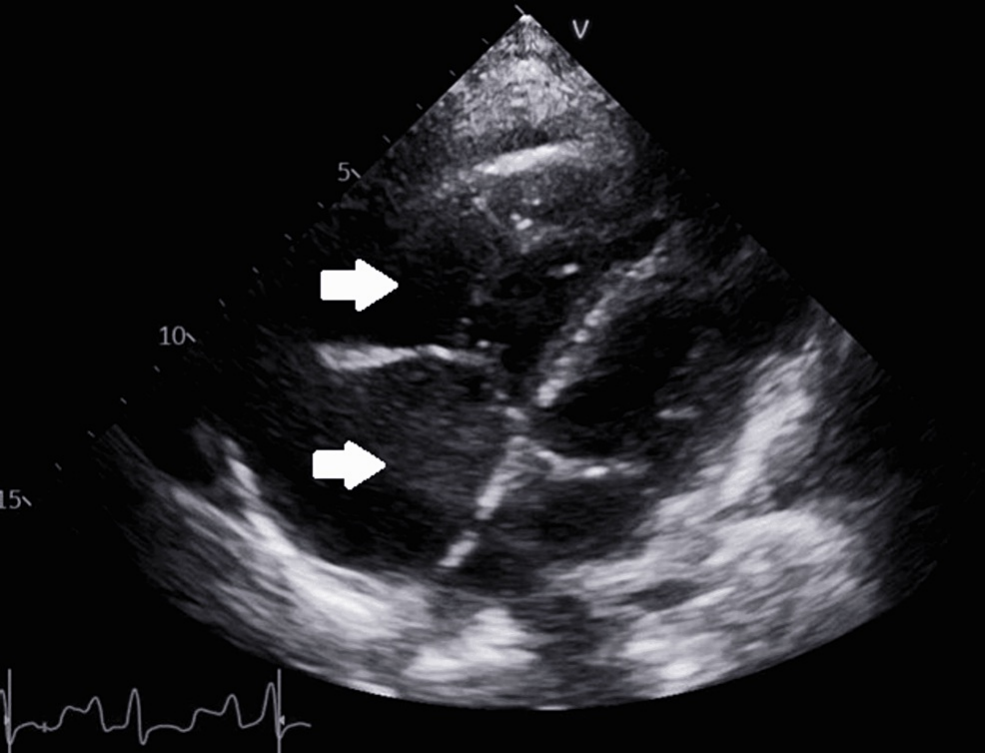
Four-chamber transthoracic echocardiogram for case 2. White arrows demonstrate an enlarged right atrium and ventricle in comparison to the left.
